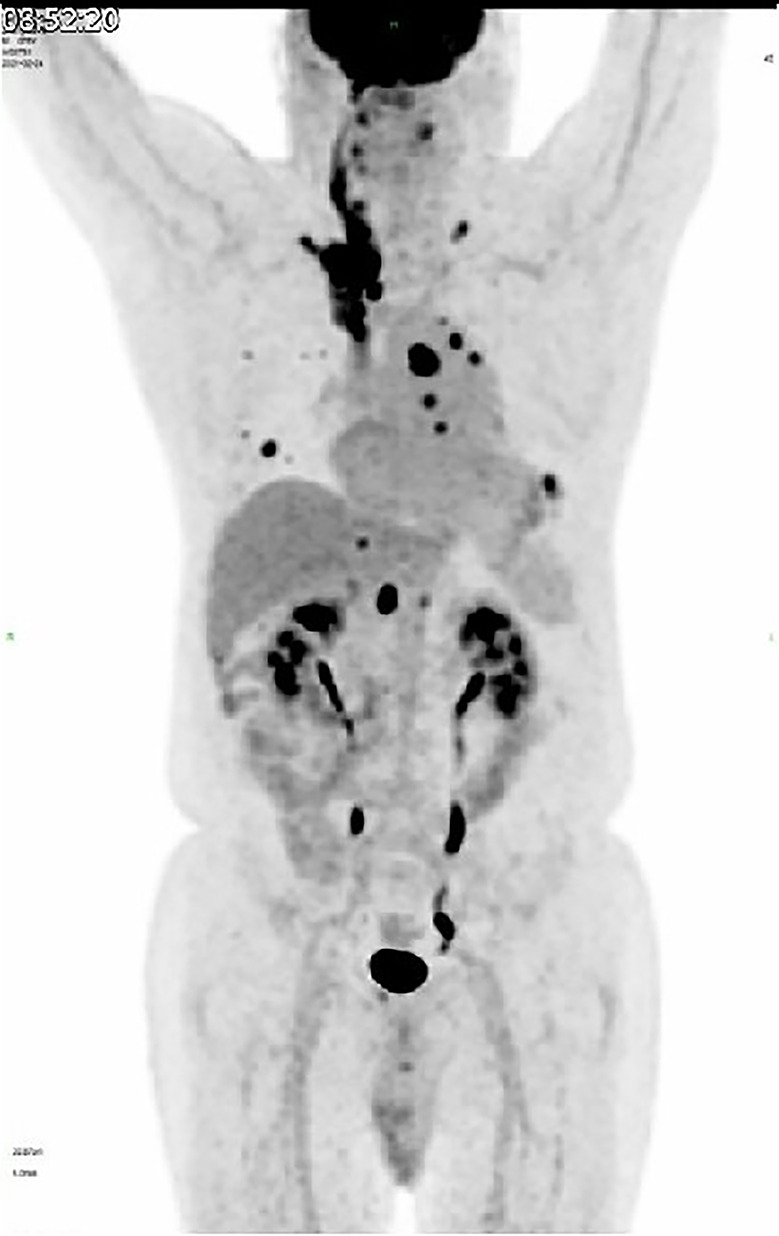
^18^F-FDG-PET scan shows hypermetabolic foci located from the origin of superior vena cava, expanding to the right cephalbrachial vein, with entrance to the right common jugular vein, right internal jugular vein, and vertebral vein.
